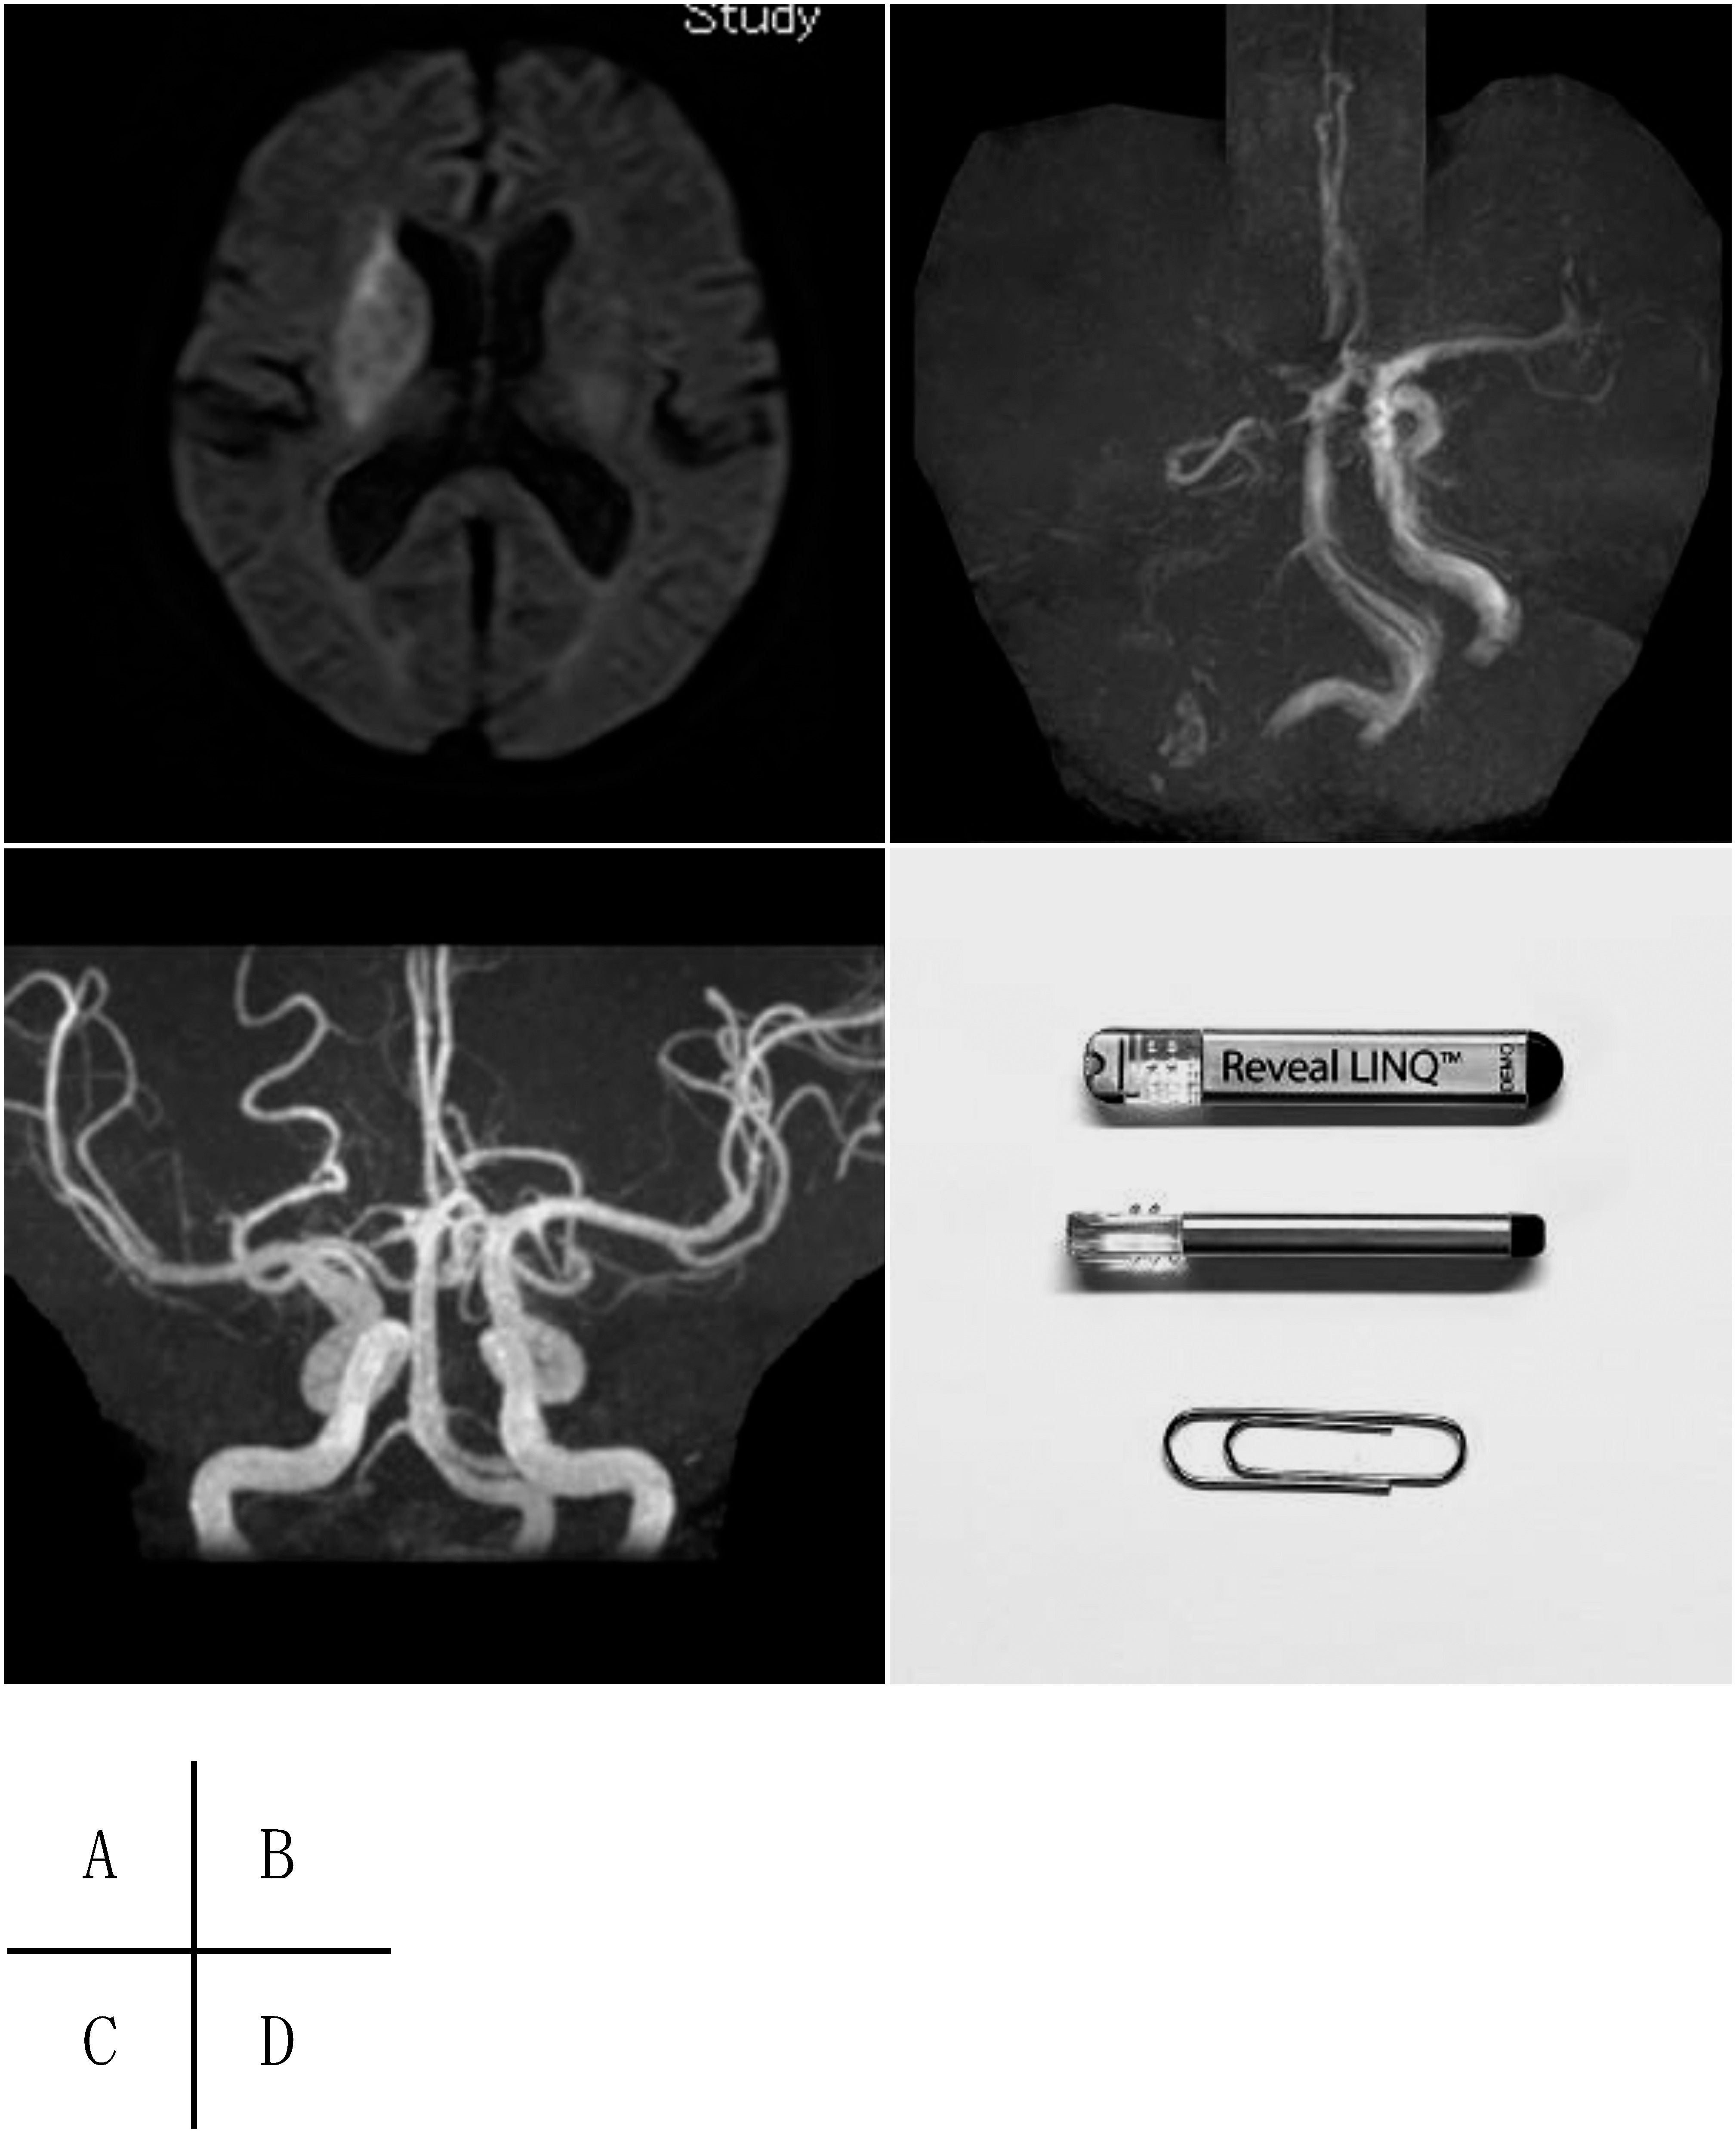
Fig. 4 A case indicating detection of PAF by ICM. (A) MRI (DWI) displayed a high-signal-intensity area in the right basal ganglia, however much of the MCA distribution appears spared. DWI-ASPECT 8/11. (B) MRA indicates right internal carotid artery occlusion. (C) Total recanalization after mechanical thrombectomy. No large vessel stenosis present on MRA at day 2. (D) ICM and paper clip. These are about the same size.
